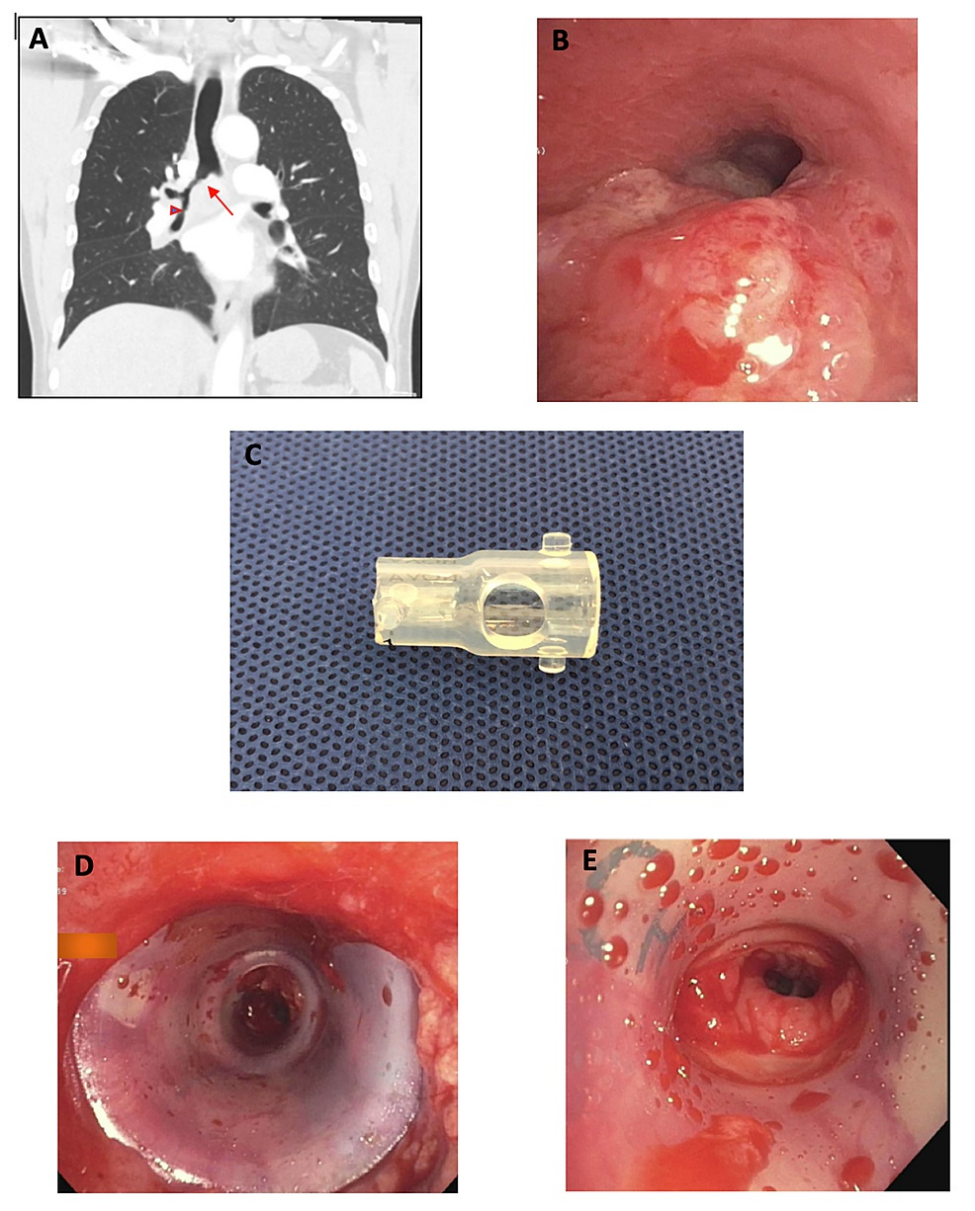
Right mainstem bronchial and right bronchus intermedius obstruction treated with modified hourglass stent A: CT demonstrating RMSB (arrow) and RBI obstruction (arrowhead). B: Bronchoscopic image of RMSB tumor causing significant narrowing of the airway. C: Modified hourglass stent cut in half with side-hole for right upper lobe bronchus. D: Inserted modified hourglass stent, with larger diameter in RMSB and smaller diameter in RBI (proximal view). E: Inserted modified hourglass stent, with larger diameter in RMSB and smaller diameter in RBI (distal view) CT: computed tomography; RMSB: right mainstem bronchus; RBI: right bronchus intermedius
